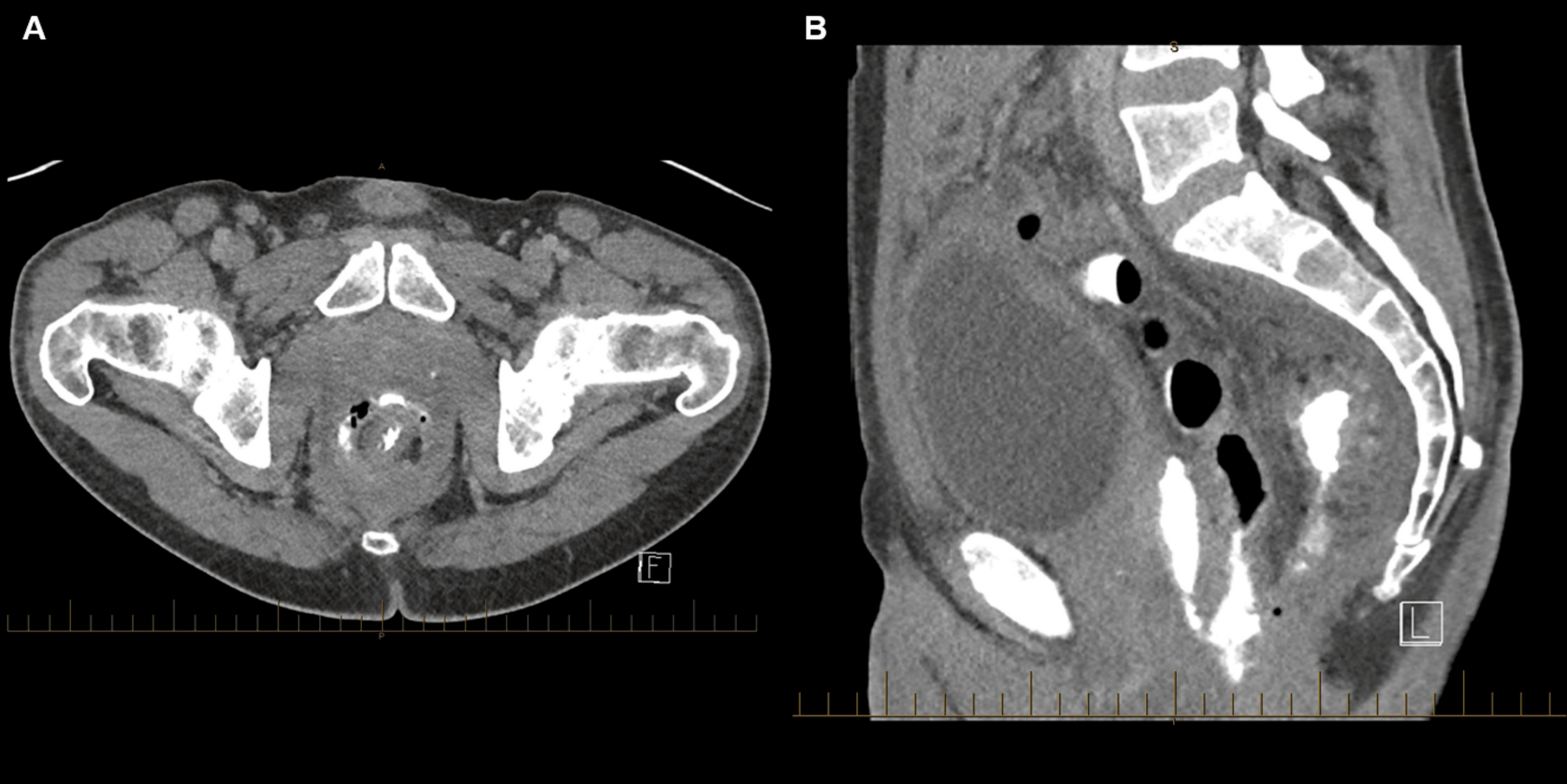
CT fistulogram of the abdomen and pelvis eighteen days after the Altemeier procedure. (A) axial, (B) sagittal. Contrast injected via the drain is visible in the collection and colon, confirming a fistula tract between the anastomosis and collection. The fistula tract is demonstrated in (B).
